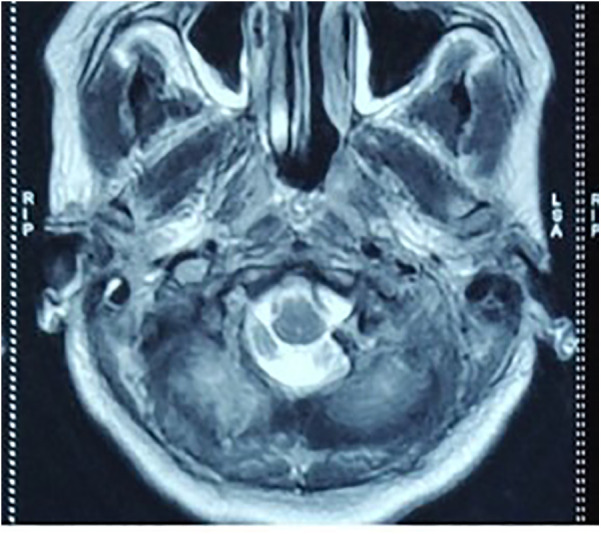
MRI of the first patient showing a lacerated fragment of the fractured occipital condyle in the foramen magnum without any neurovascular compression.
